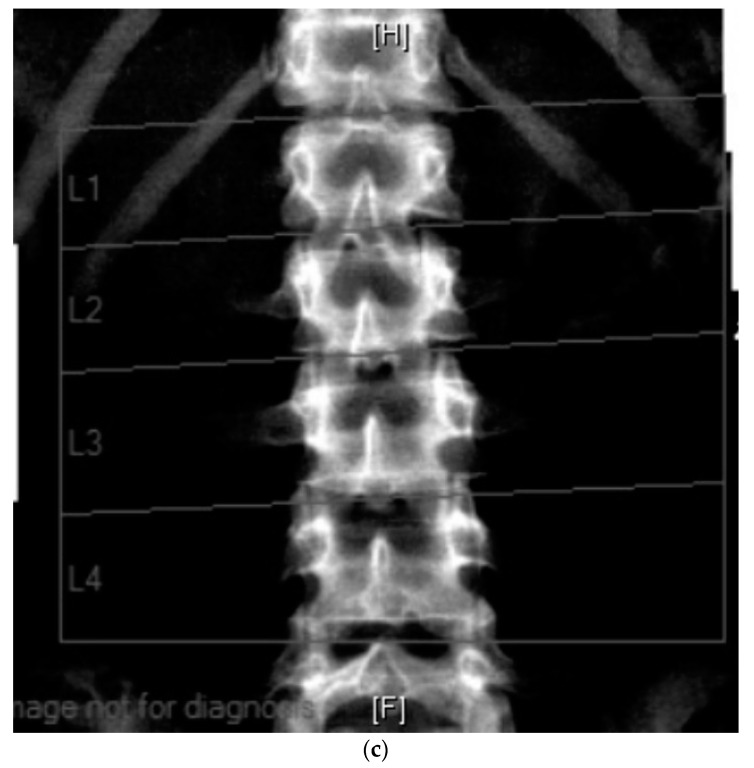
(a) Anterior posterior view—whole spine x-ray in a 25-year-old female patient affected with PMM2-CDG; bone mineral density is below expected for her age (T score −2.6, z-score −2.6); (b) lateral view; (c) radiological features of her lumbar spine: there is a double mild curve scoliosis and no rotational deformity. All pedicles are visualized. There is impression of slight upper hyperkyphosis and impression of multilevel Schmorl’s nodes.
